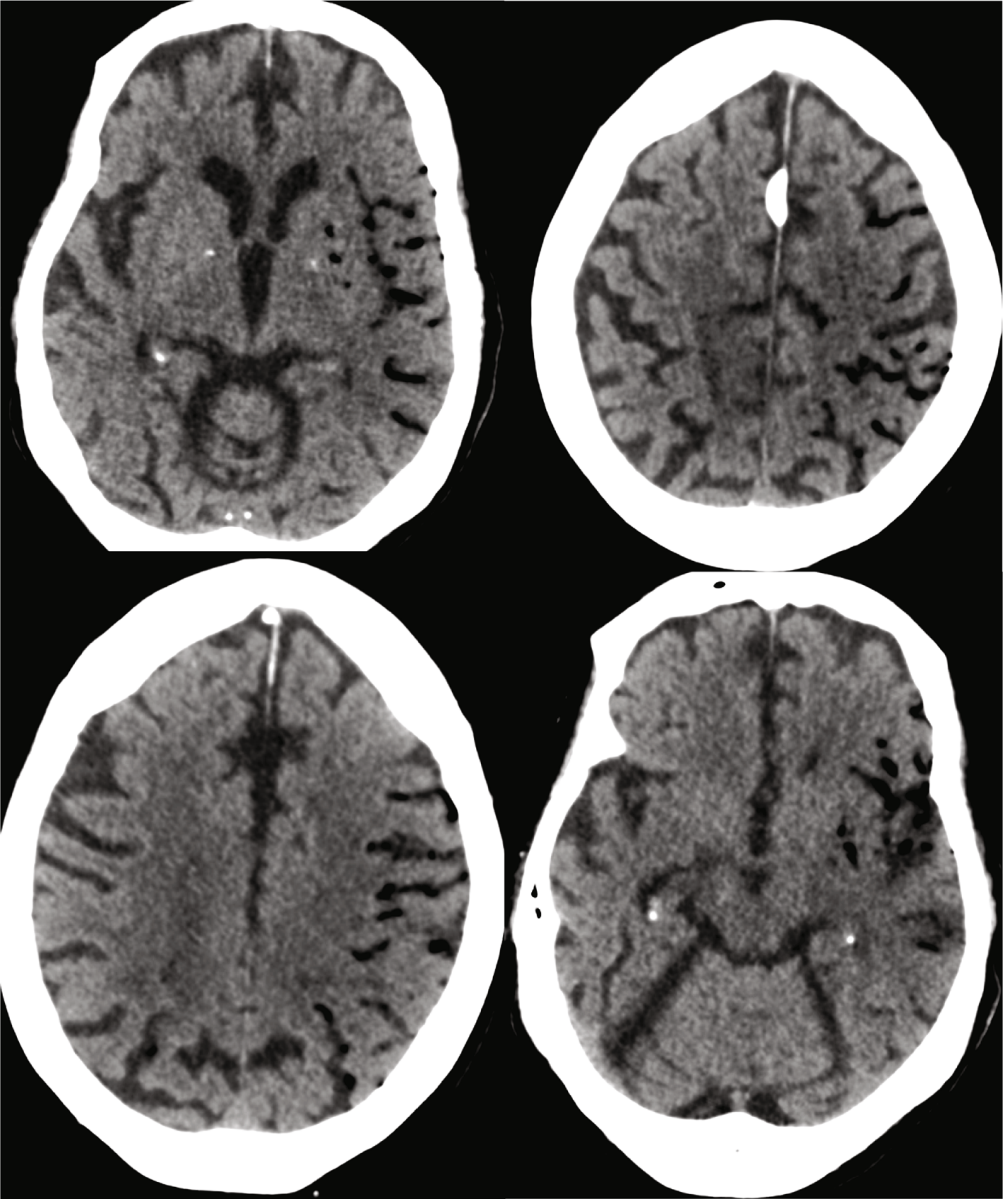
Multiple axial CT images of the head without contrast obtained after the patient's abrupt mental status changes demonstrate an extensive amount of intravascular air within the left MCA branches.
